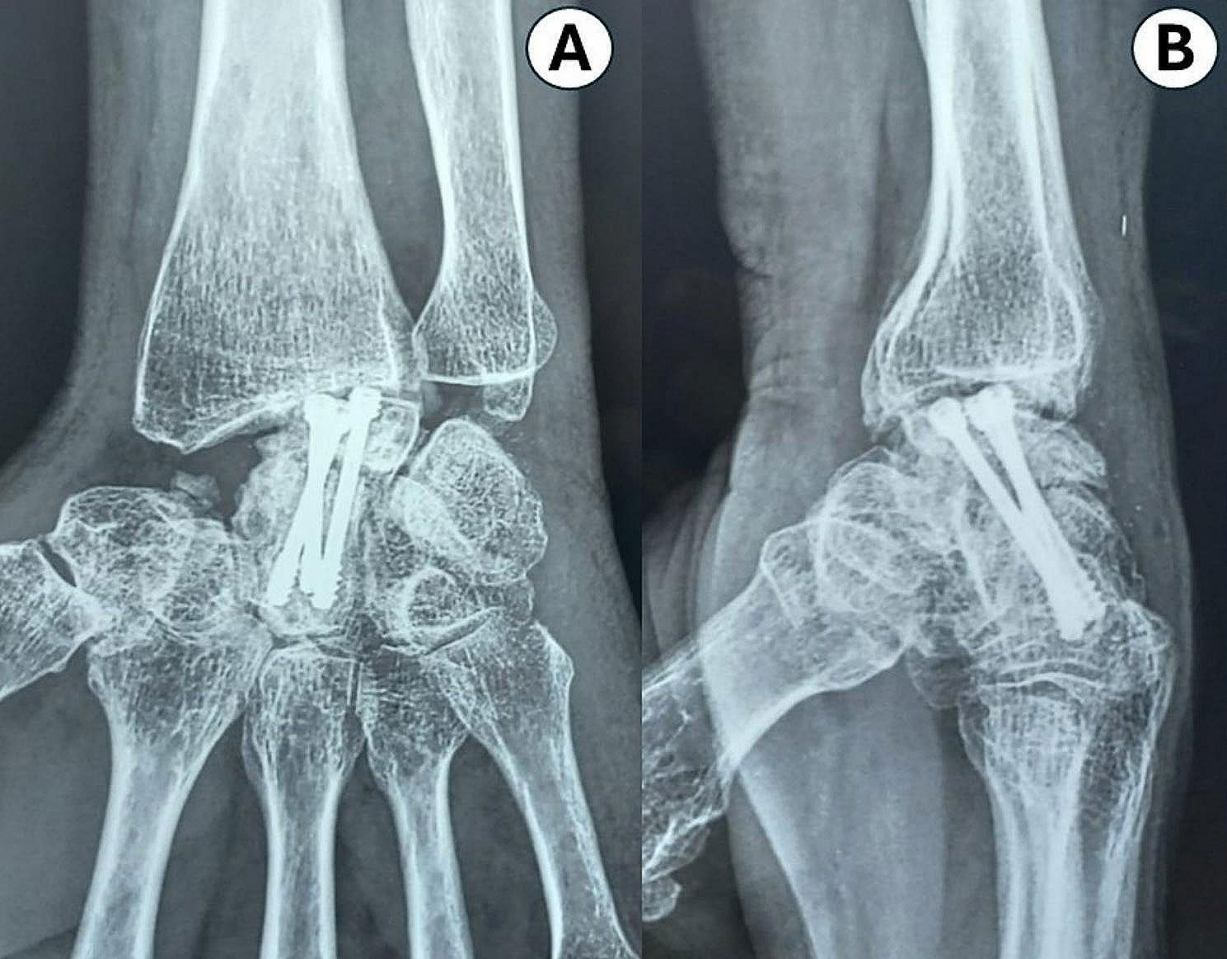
A: Anteroposterior, and B: Lateral wrist radiographs demonstrating RL osteoarthritis with backed out screws of the lunate following LCF with antegrade inserted Herbert screws
